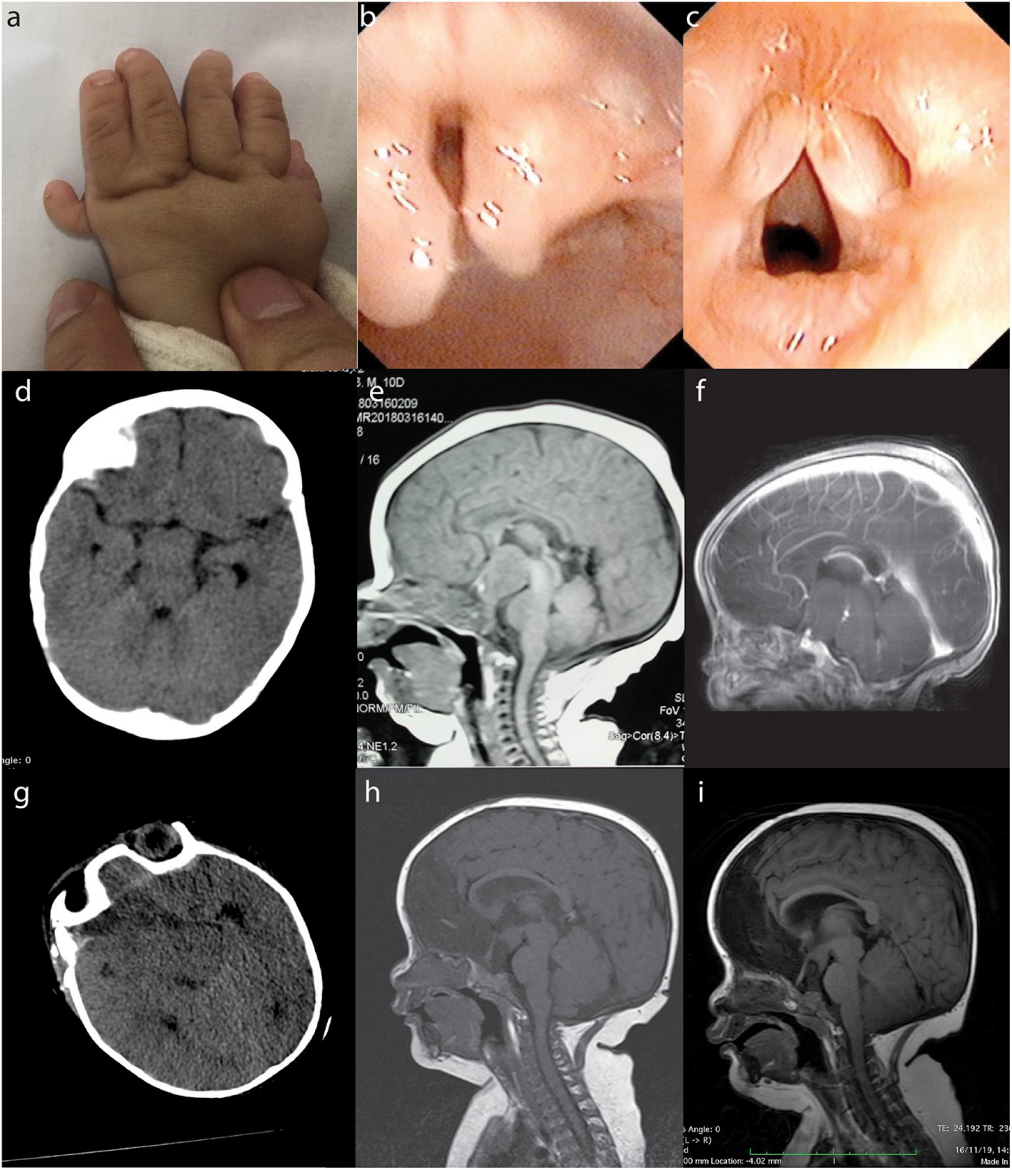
PHS in a 7-month-old boy. The patient presented with typical morphological features of PHS such as polydactyly and syndactyly (a: left hand), bifid epiglottis (b), and laryngomalacia (c). Preoperative CT (d: at 7 months of age), non-contrast MRI (e: 12 days postnatal), and contrast-enhanced MRI (f: 7 months of age) showed a suprasellar lesion. Postoperative CT (g) and MRI (h) revealed subtotal removal of the lesion, and follow-up MRI 1 year later showed no tumor regrowth (i). PHS, Pallister–Hall syndrome; CT, computed tomography; MRI, magnetic resonance imaging.
